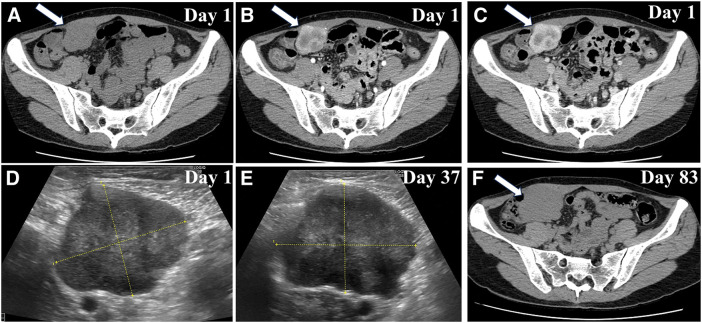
Imaging findings (A) Contrast enhanced CT on February 7. A 40 × 35 mm mass was identified in contact with the right lower abdomen. (B and C) Early enhancement was observed at the margins, with delayed enhancement within the interior. (D) Abdominal US on February 7. A hypoechoic mass (41.8 × 35.2 mm) was observed in the right lower abdomen. The mass had a lobulated shape, with a mix of hyper- and hypoechoic areas internally. (E) Abdominal US findings on March 15 revealed that the mass had increased in size by 9 mm in the long axis and 7 mm in the short axis over 1 month. (F) Plain CT on April 30, showing that the mass had increased to 61.3 × 53.1 mm. US, Ultrasonography
